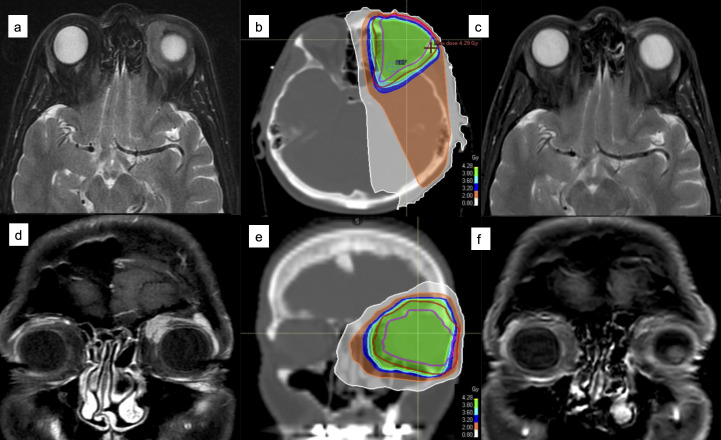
A 55-year-old lady diagnosed with stage I ocular MALT (a, d) was treated with LDRT (b, e) in a curative setting. She obtained CR at the first MRI revaluation (c, f) and is still well being and in complete remission 1 year after radiotherapy.
